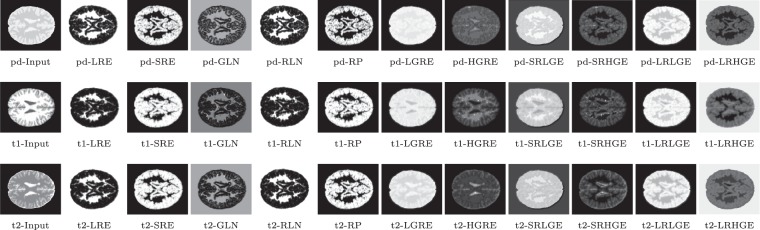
Input MRI images of three pulse sequences (PD, T1, and T2) and 11 features extracted for each.
